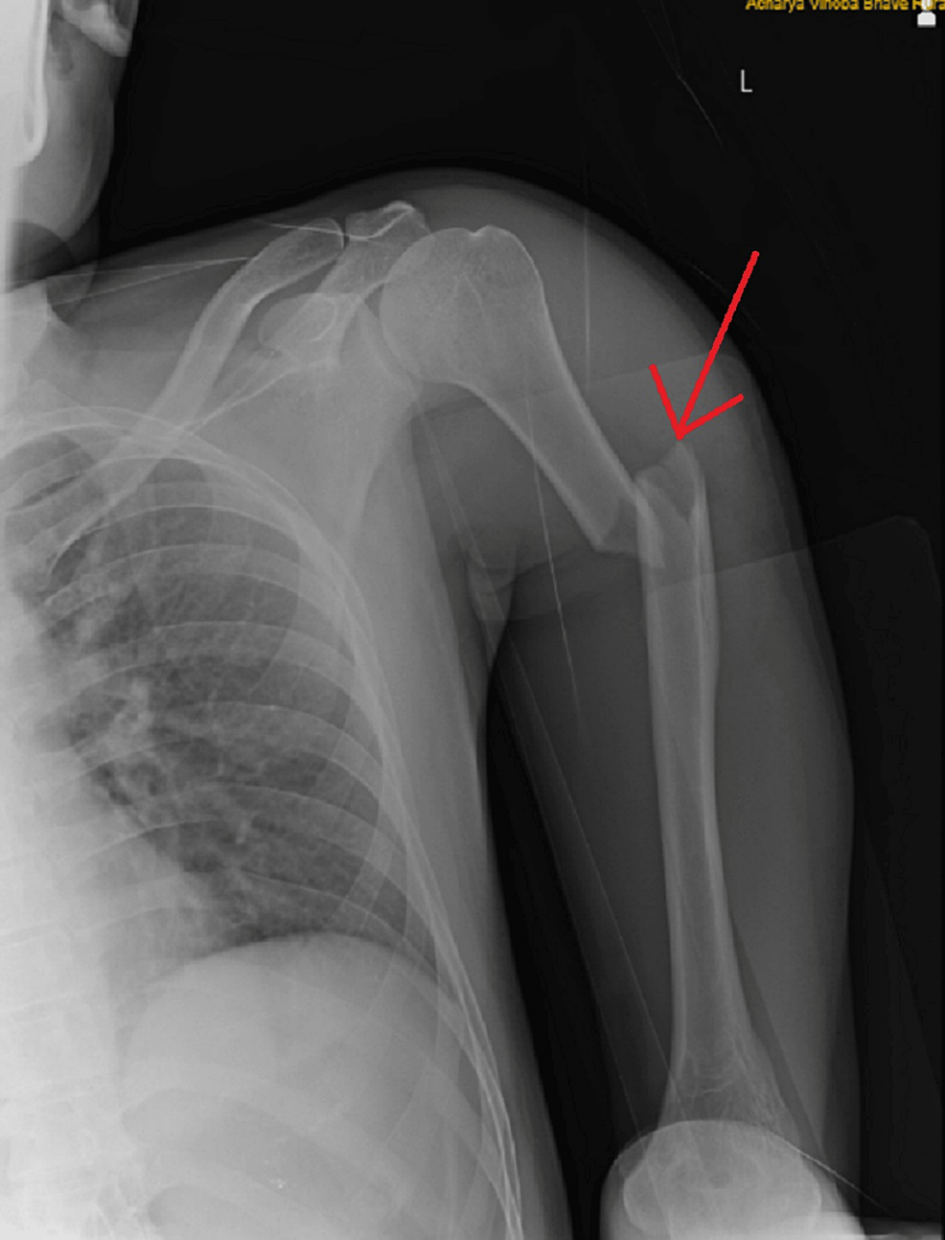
Anterior-posterior radiograph showing short oblique fracture in proximal humeral shaft region on left side
